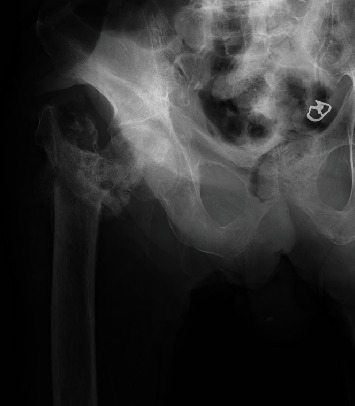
A radiograph at 1 year after implant removal showing nonunion of the right trochanter of the femur.
